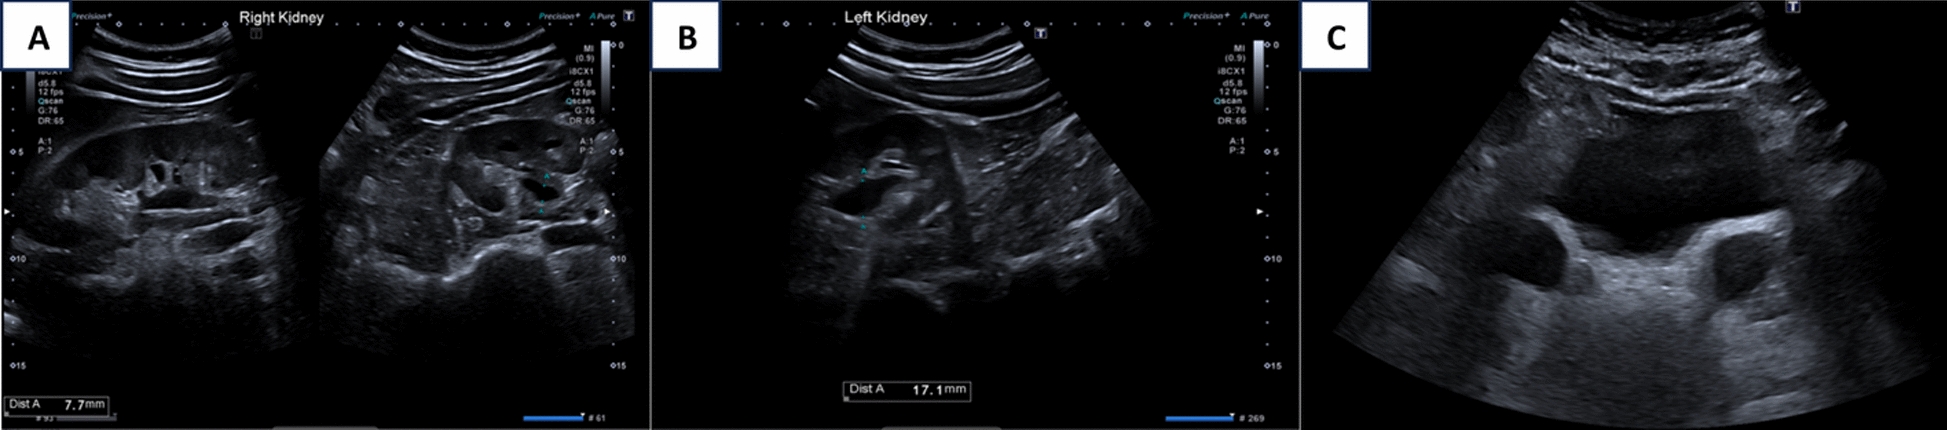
The ultrasound images of the kidneys and bladder. In A, the right kidney shows hydronephrosis with a renal pelvis measuring 1.5 cm in anteroposterior diameter. After emptying the bladder, the anteroposterior diameter decreased to 0.7 cm. In B, the left kidney shows mild hydronephrosis with a renal pelvis measuring up to 1.7 cm in anteroposterior diameter. After emptying the bladder, the anteroposterior diameter of the pelvis did not change. In C, the bladder is well distended and demonstrates no focal or diffuse wall thickening or trabeculations. In addition, there is bilateral distal ureteral dilatation measuring 2.5 cm on the right side and 1.8 cm on the left side, which did not resolve even after emptying the bladder
